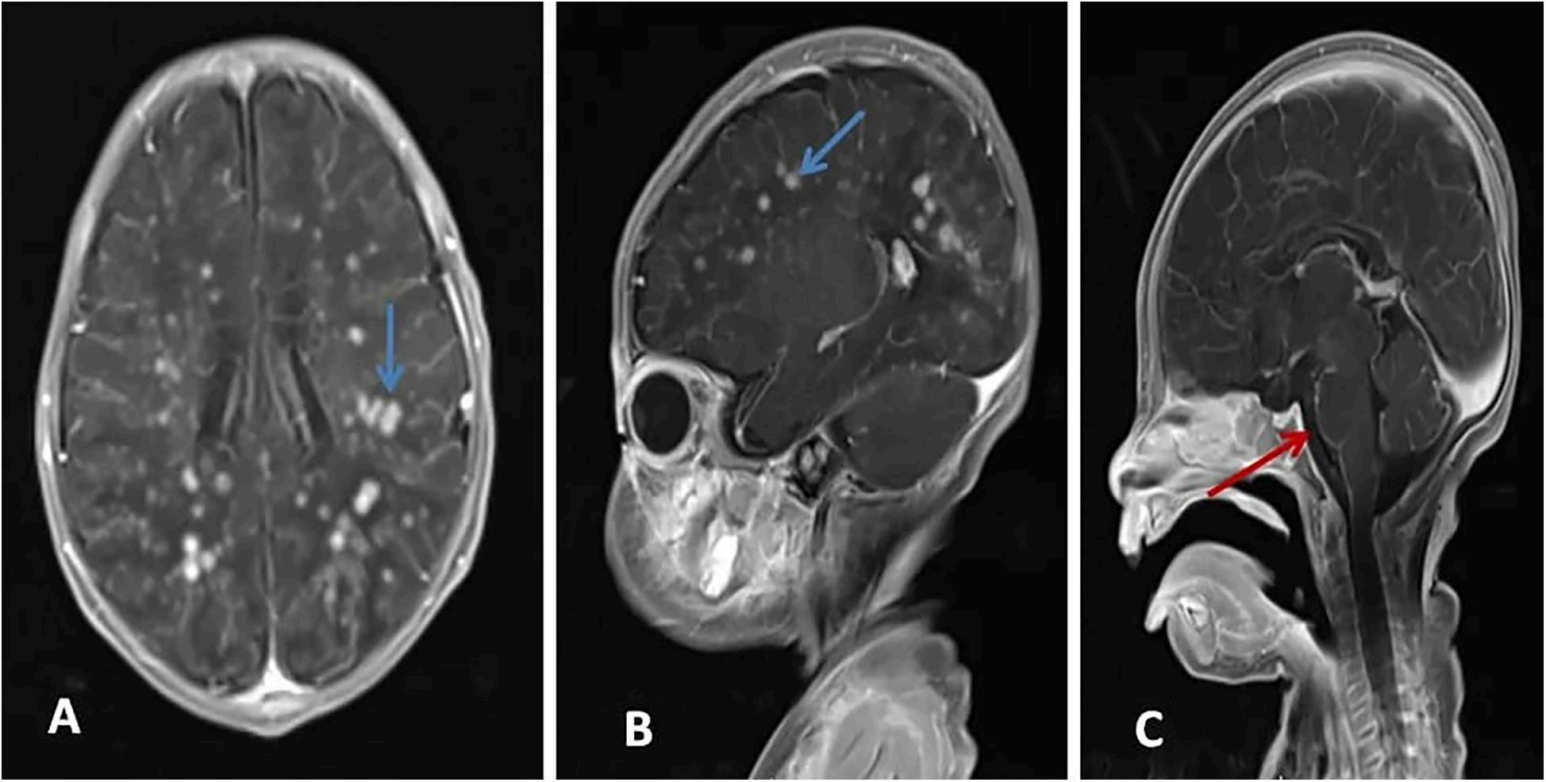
Axial (A) and sagittal (B,C) views of post-contrast MRI show avid nodular enhancement (blue arrows) and leptomeningeal enhancement (Red arrows).
